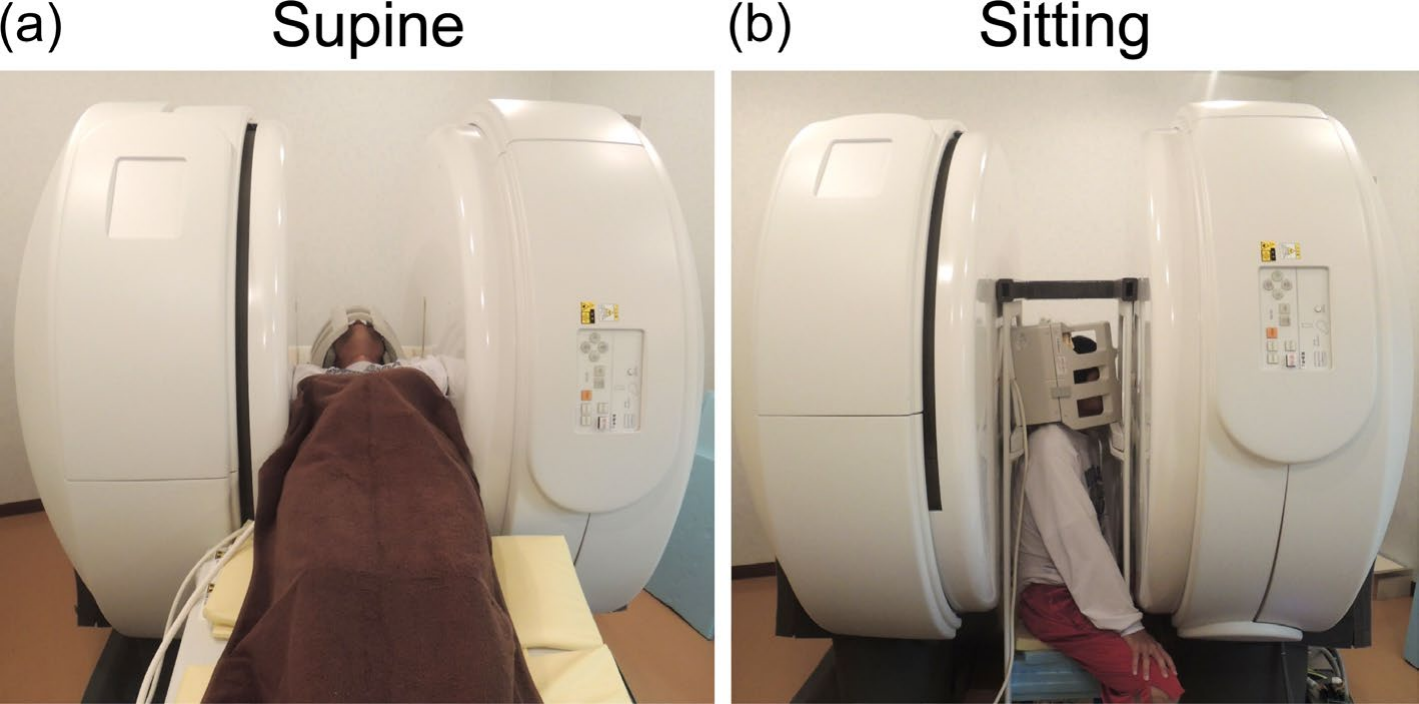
(a) Supine and (b) sitting positions in multi‐posture MRI.
